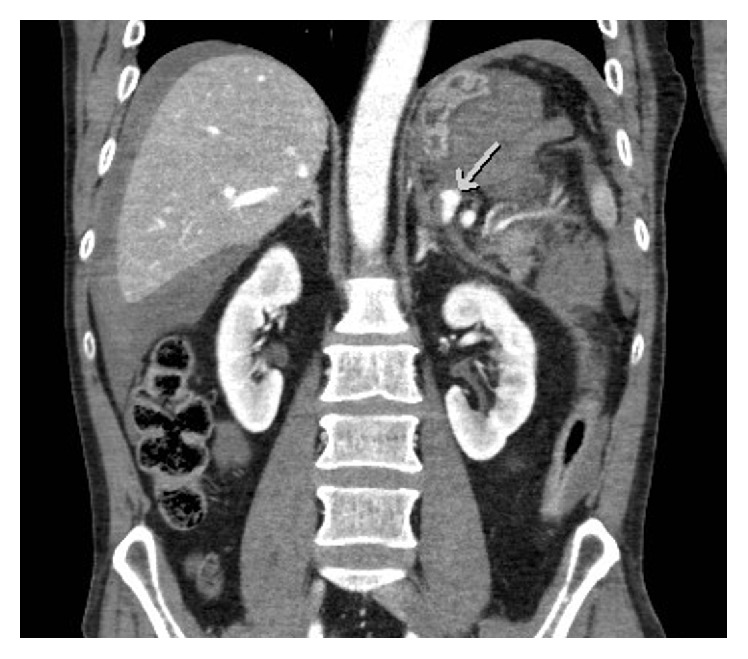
9 mm contrast-filled dilatation of splenic artery (arrow) on initial CT.
